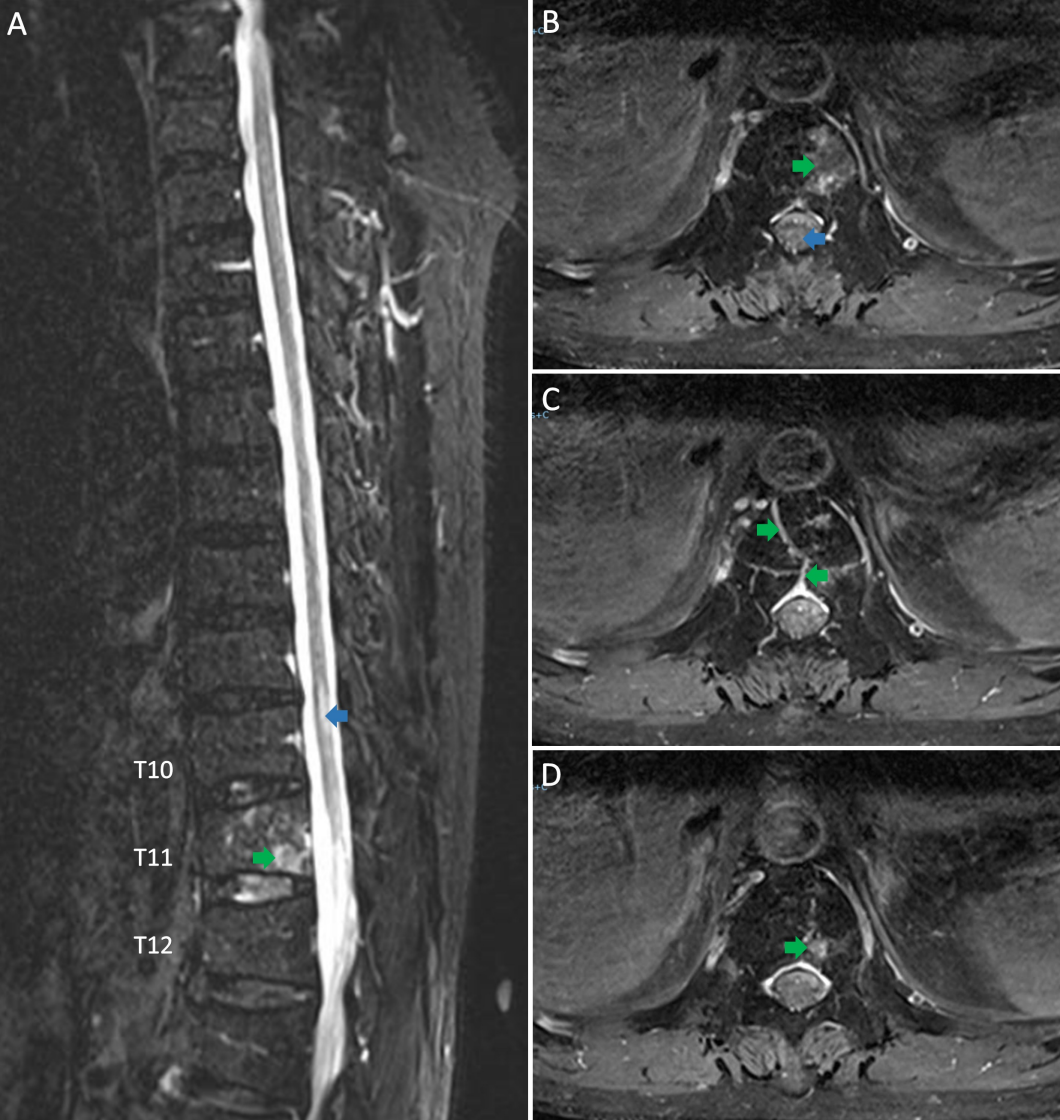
The repeat T2-weighted magnetic resonance imaging of the thoracic spine two weeks later. A: the sagittal image and B, C, D: the axial images showing central hyperintensity of the spinal cord (blue arrows) and abnormal signal within the T11 vertebral body (patchier enhancement shown by green arrow as compared to Figure 1) and the spinal cord indicated by blue arrows (less pronounced as compared to Figure 1).
